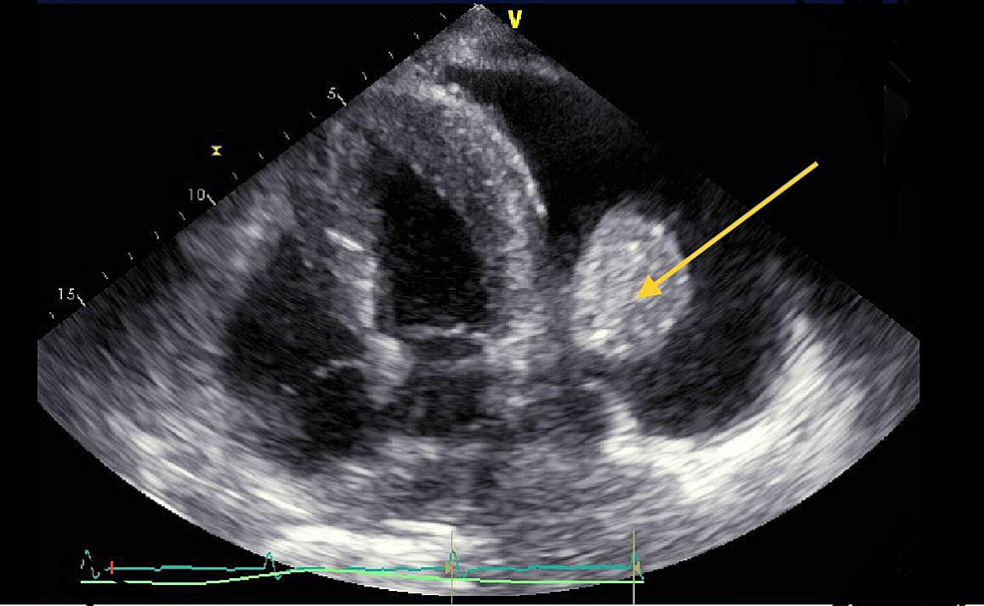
Apical four-chamber view on transthoracic echocardiogram showing mass extending from the pleura (yellow arrow).
